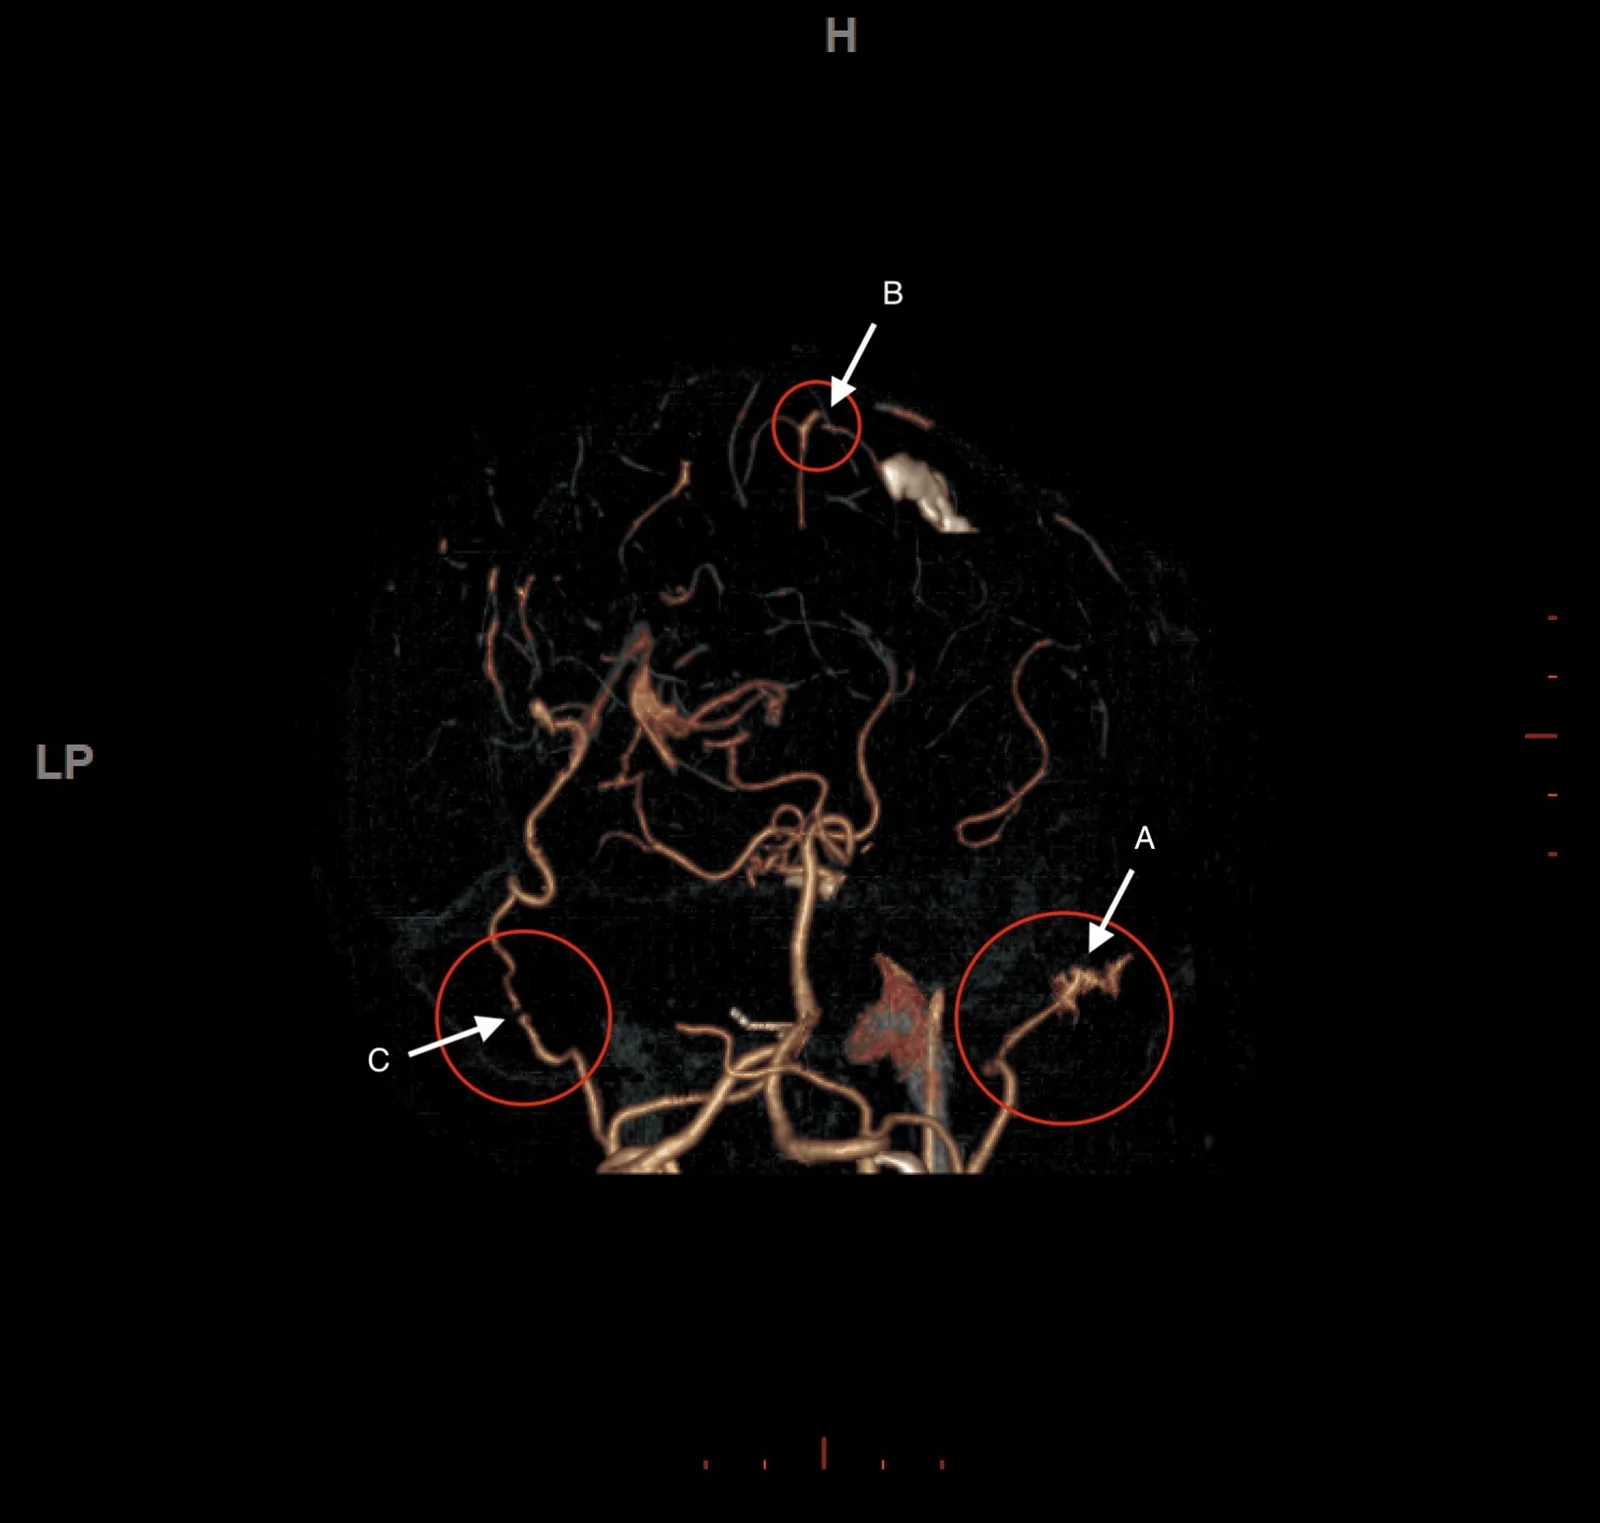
CTA of the head demonstrating characteristic moyamoya collaterals and progressive arterial stenosis A: The characteristic 'puff of smoke' appearance associated with MMD, indicative of the formation of fragile collateral vessels due to steno-occlusive changes in the ICAs. B and C: Varying degrees of stenosis observed within the arterial system, highlighting the pathological narrowing of vessels contributing to the progressive nature of MMD. CTA: Computed tomography angiography, MMD: Moyamoya disease, ICAs: Internal carotid arteries
